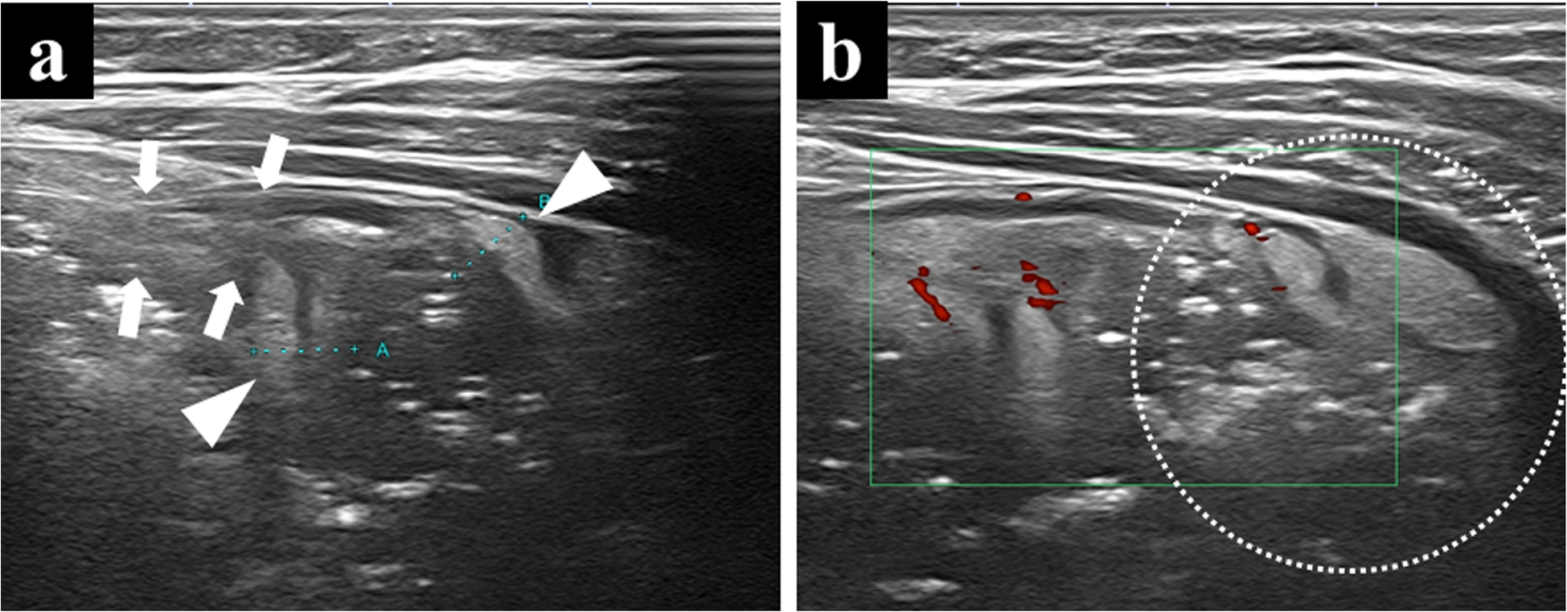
Abdominal ultrasonic findings of the intestinal lesion with a high-frequency 11-MHz linear transducer. a shows the poorly deformable intestinal tract, which narrows rapidly (arrow) from the dilated segment (arrowhead) and has a thin wall with irregular laminar structure; b shows the peripheral side of the focally narrowed intestinal tract that has no power Doppler signal enhancement and no “creeping fat sign” around the lesion
